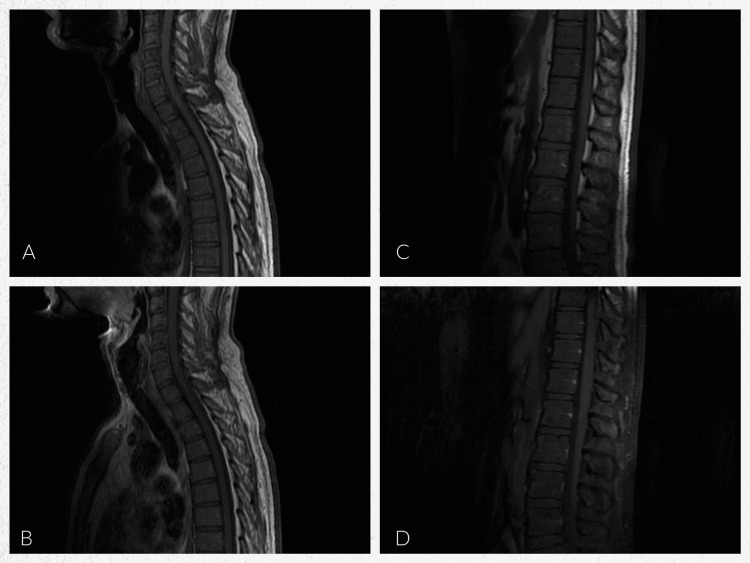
MRI cervical, thoracic, and lumbar spine with and without contrast A: MRI cervical and thoracic spine, sagittal view, T1 without contrast. B: MRI cervical and thoracic spine, sagittal view, T1 contrast enhanced. C: MRI thoracic spine and lumbar spine, sagittal view, T1 without contrast. D: MRI thoracic spine and lumbar spine, sagittal view, T1 fat-suppressed contrast enhanced. Imaging of the cervical spine showed no suspicious pathologic bone marrow replacement in the cervical vertebrae and no evidence of acute compression fracture, paraspinal mass, or enhancing intramedullary or epidural mass lesions. No evidence of a syrinx. Imaging of the thoracic spine showed no evidence of thoracic cord enlargement or suspicious enhancing intramedullary or epidural mass lesion and no evidence of midline or foraminal herniation or cord compression. Imaging of the lumbar spine showed no evidence of suspicious signal intensity changes within the cauda equina, no evidence of pathologic bone marrow replacement in the lumbar vertebrae, no acute compression fracture, and no paraspinal mass. There was no evidence of enhancing intramedullary or epidural mass lesions or spinal stenosis. Permission to reproduce obtained from the St. John's Riverside Hospital Department of Radiology and reviewed by co-author, Dr. Farid Abdo.
